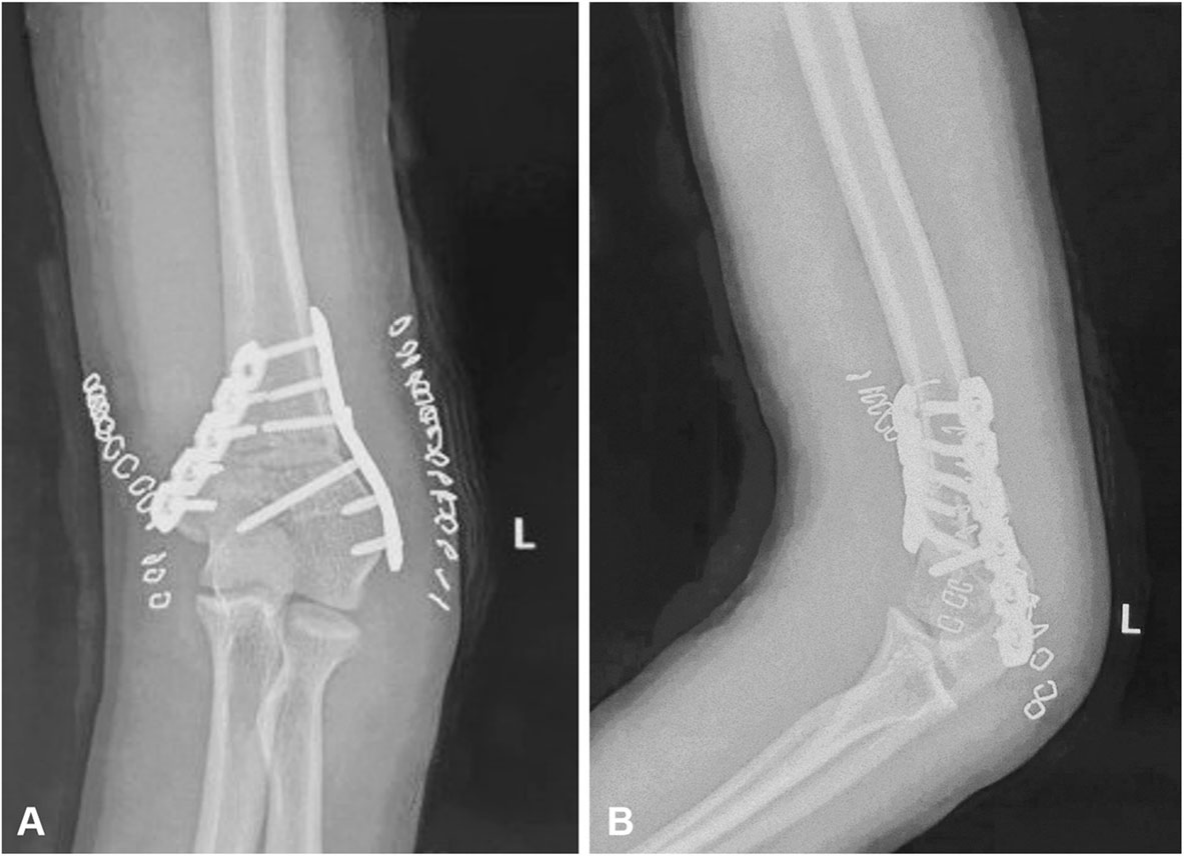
Radiographs after the operation. a Positive elbow joint X-ray. b Lateral elbow joint X-ray
